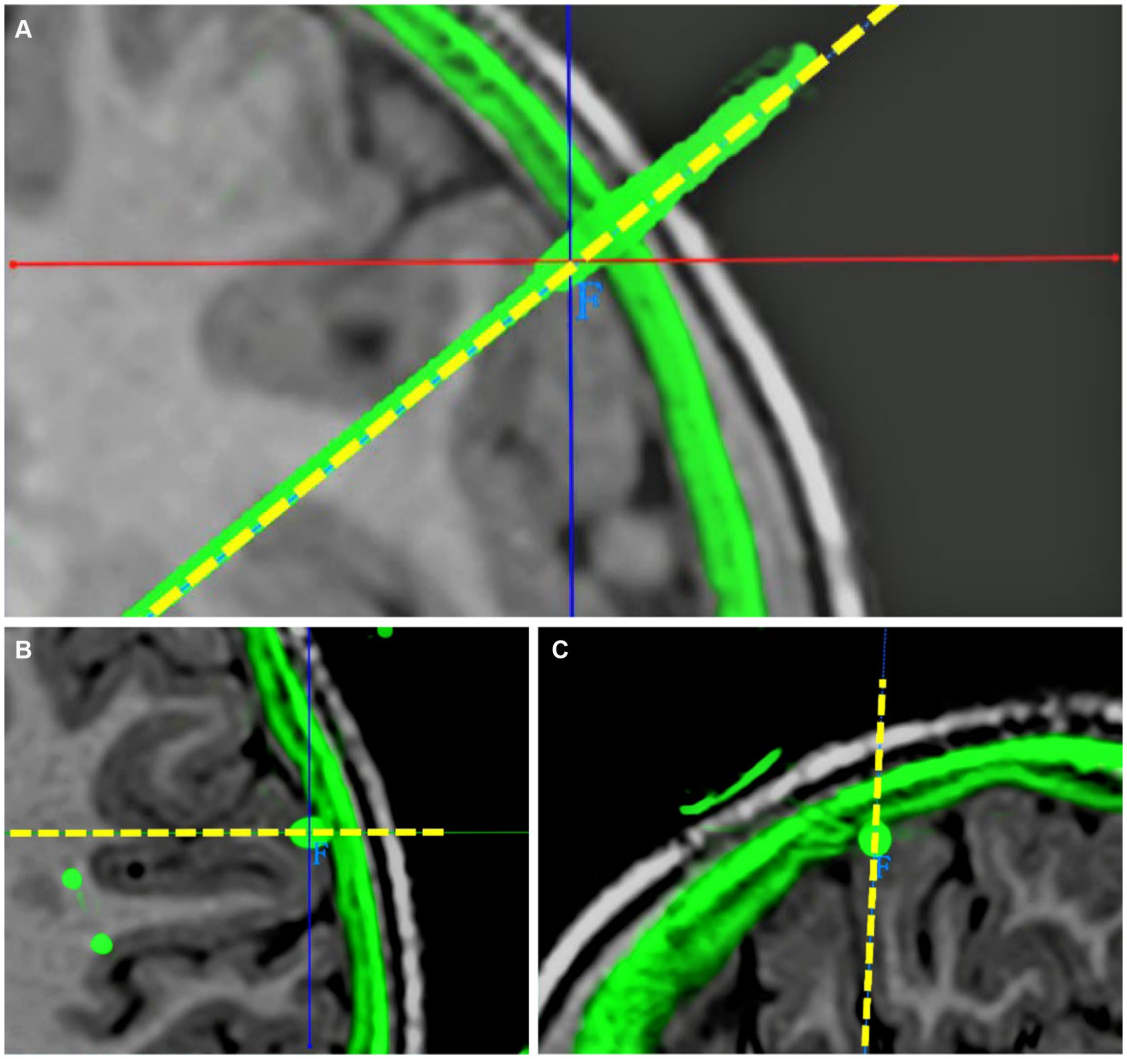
Determination of EPLE. (A) Coronal visualization of planned and actual electrode trajectories. (B) The planned and actual electrode tracks are displayed in the transverse plane. Their entry points coincided. (C) The planned and actual electrode tracks are shown in the sagittal plane. Their entry points coincided. The junction of solid red and blue lines is the center of the actual electrode track entry point, the dotted yellow line is the planned electrode track, and the green part outside the cerebral cortex is the skull shadow in the postoperative CT after registration. The EPLE is 0.31 mm.
